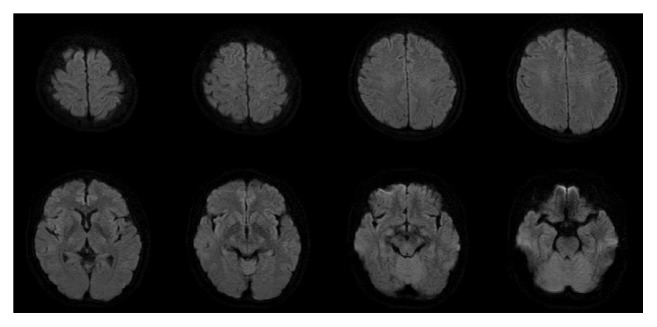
Diffusion-weighted images (DWIs) of MRI on the next day of admission were normal.
